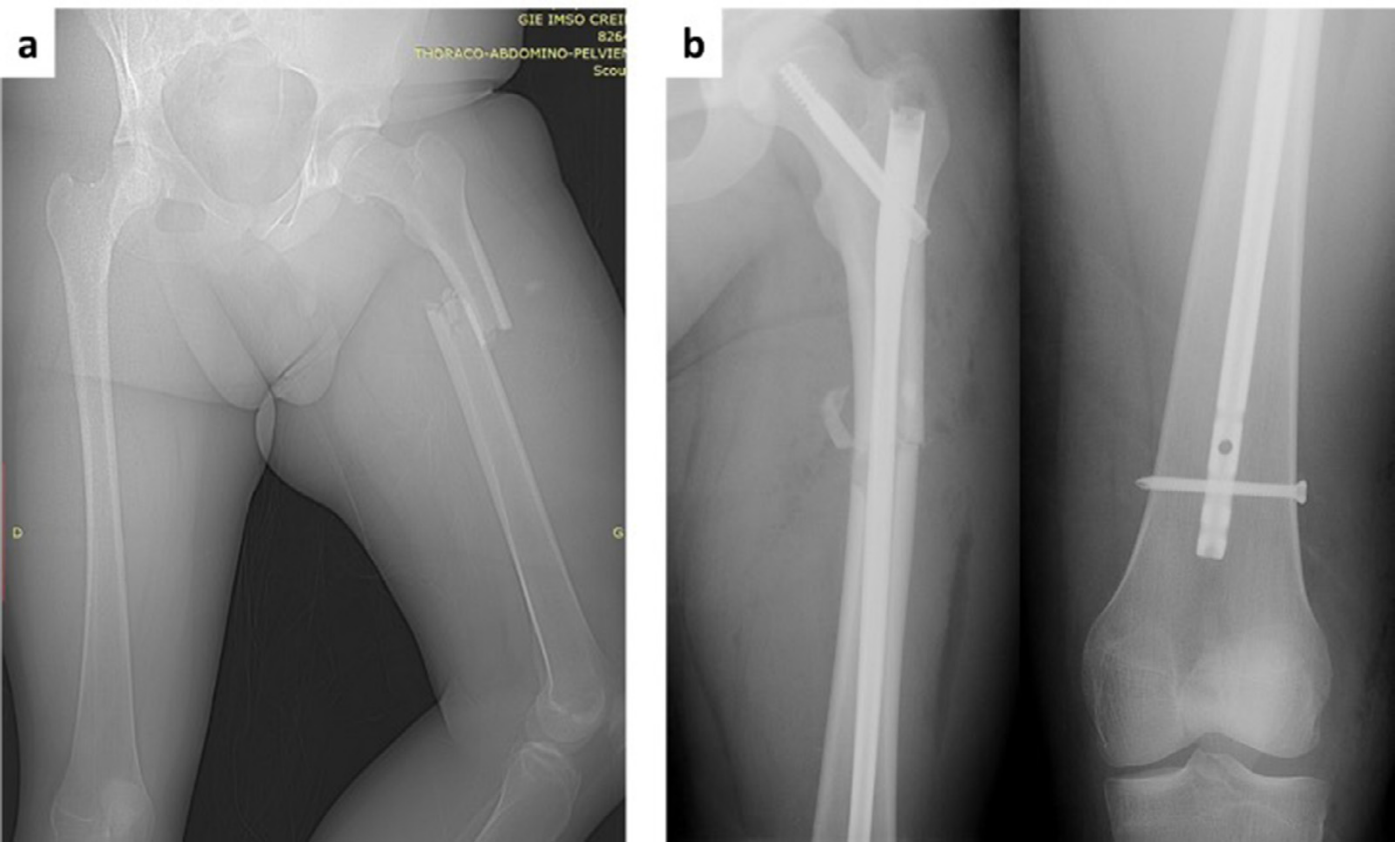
(A) Fracture of the proximal third of the left femoral shaft on computed tomography examination (scout). (B) Osteosynthesis with a long Gamma 3 nail (Stryker, Pusignan, France).
